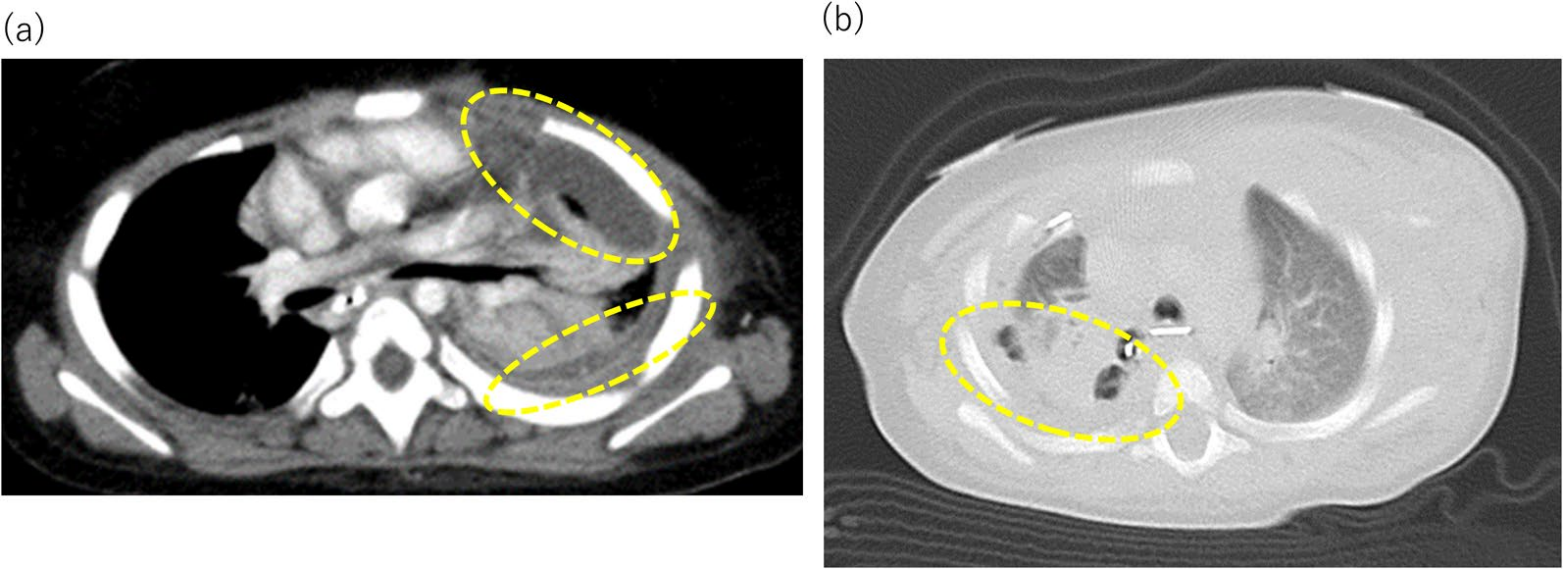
Preoperative computed tomography scans of the chest in Cases 1 (a) and 2 (b) show a large amount of air-containing fluid collection with loculation in the left and right pleural space (dotted circle), respectively
